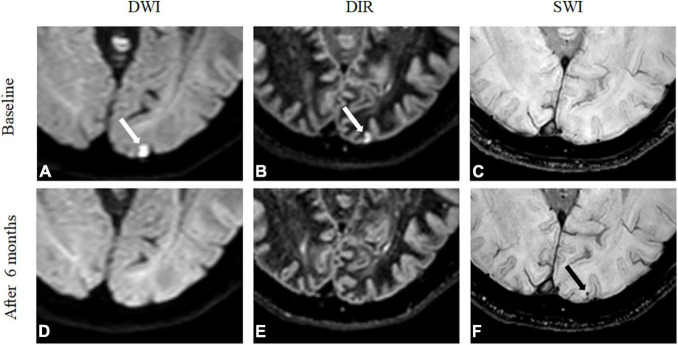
De novo* cerebral microbleeds matched with microinfarcts. A 71-year-old woman showed an embolic microinfarct that was detected in the left occipital lobe on DWI (A) and 3D-DIR (B) at baseline, but no abnormality was found on SWI (C). After 6 months, DWI (D) and 3D-DIR (E) did not detect an abnormality. A *de novo* CMB was detected in the left occipital lobe by SWI (F), and the lesion was in the same location as where the embolic infarction was detected on baseline MRI.
